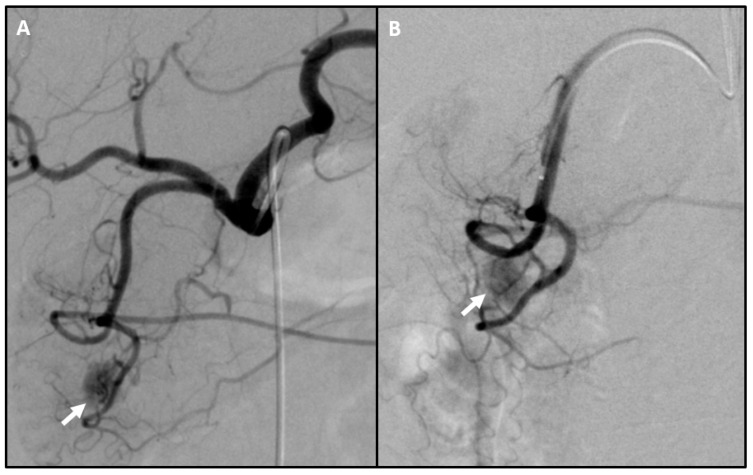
Hypervascular insulinoma on arteriogram. (A) Celiac arteriogram after selection with a Sim 1 catheter revealing conventional anatomy and a hypervascular insulinoma (arrow) in the territory of the gastroduodenal artery. (B) Sub-selective gastroduodenal arteriogram with a Progreat catheter confirms hypervascular insulinoma (arrow). No corresponding enhancing lesion was present on pre-procedural CT or MRI.
